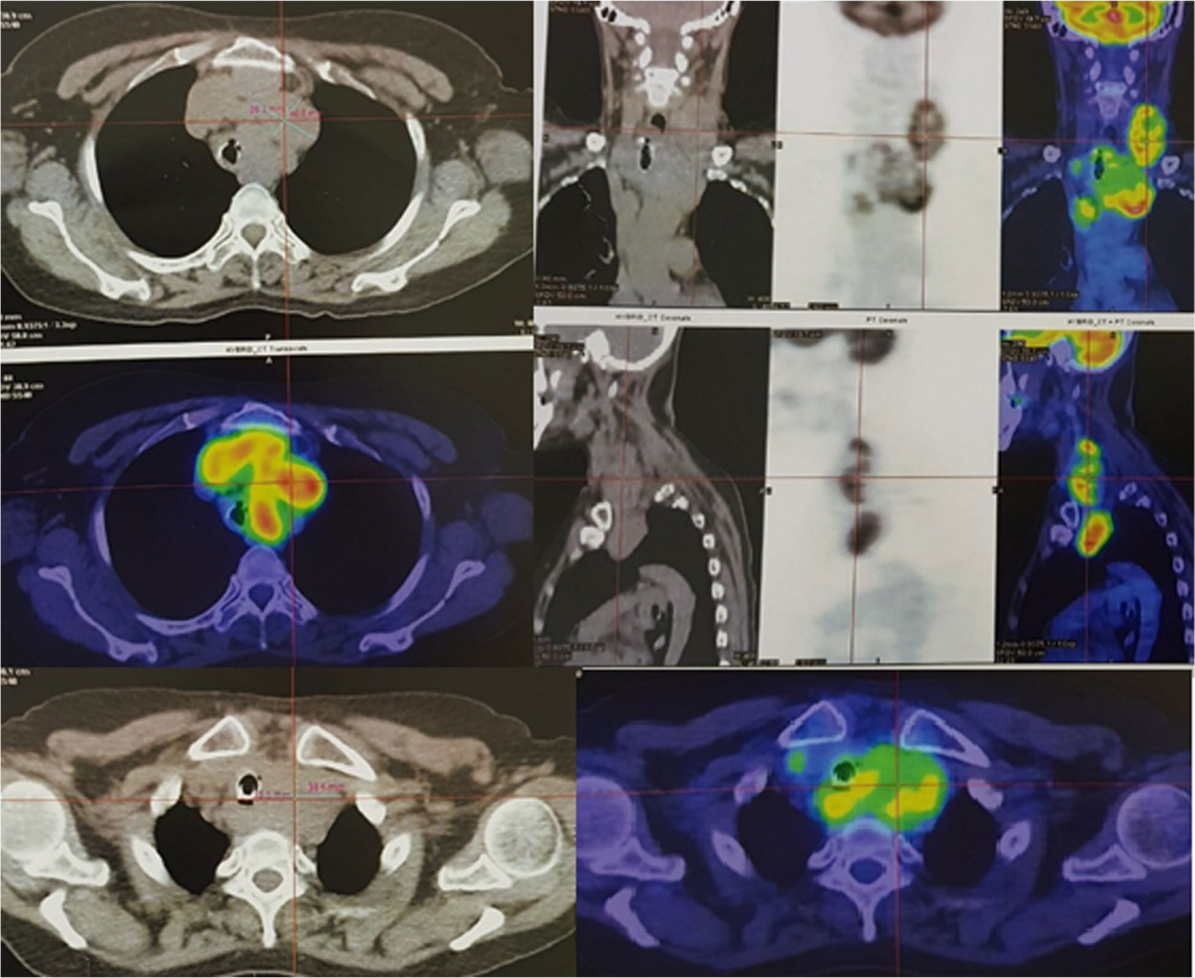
PET/CT: A 33 × 39x49 mm mass on the left side of the anterior mediastinum; multiple lymph nodes near the lower trachea, next to the superior vena cava, posterior
